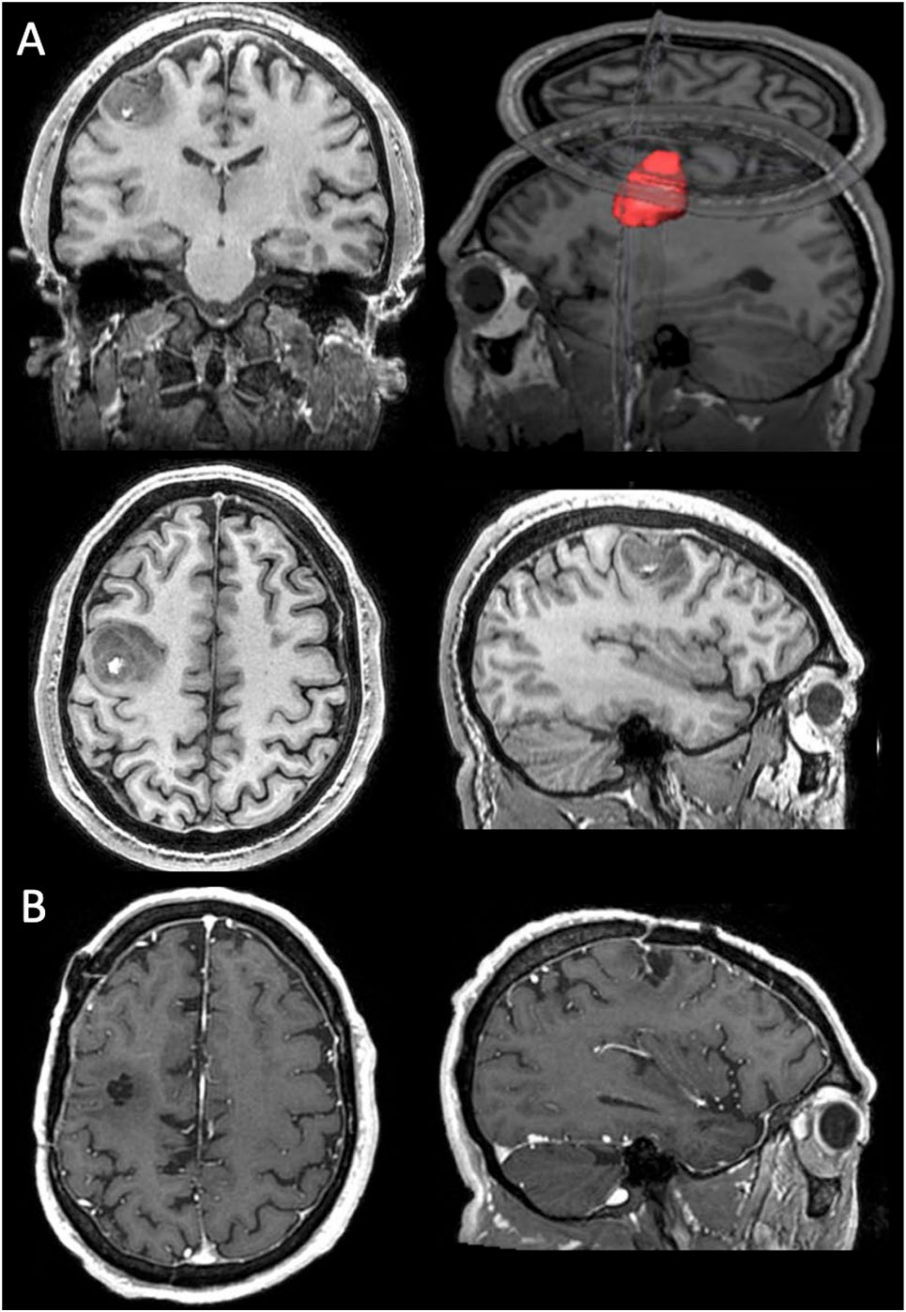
Patient AM’s T1-weighted magnetic resonance imaging scans before and after surgery. A T1-weighted (SPGR) magnetic resonance imaging scan in native space showing lesion location in the left posterior prefrontal and precentral cortex at the level of the hand region. Top right panel shows a 3D reconstruction of the lesion volume. Top left, bottom left and bottom right pictures represent coronal, transverse and sagittal slices centered on the lesion volume, respectively. B Transverse and sagittal views of the post-surgery MR scan (with T1 enhancement after gadolinium administration) in native space, coregistered to the pre-surgery scan
